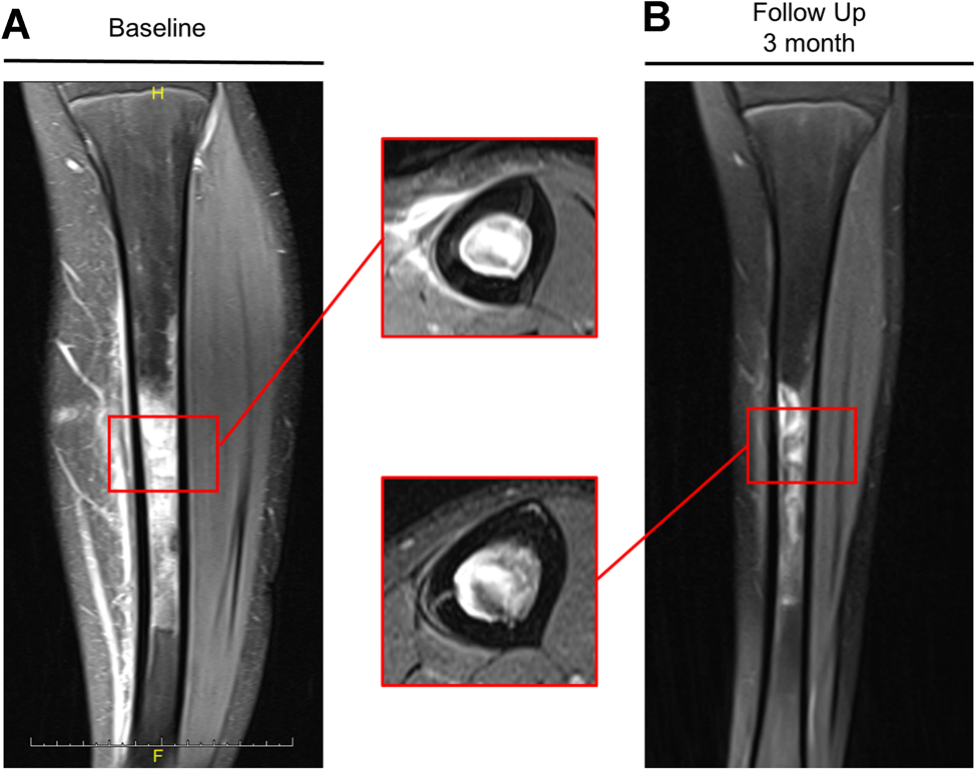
Coronal T2 MR images of a 14‐year‐old female patient at (*A*) initial presentation and (*B*) at follow‐up after 3 months demonstrating bone marrow edema of the tibia diaphysis. The patient was an active handball player and suffered from persistent pain at the tibia. She was first diagnosed with osteomyelitis and treated with antibiotics until transferred to our institution. Laboratory assessment revealed low alkaline phosphatase activity and elevated pyridoxal‐5‐phosphate levels. TNSALP mutation analysis showed a heterozygous mutation (c.746G>T) and the patient was diagnosed with hypophosphatasia.
